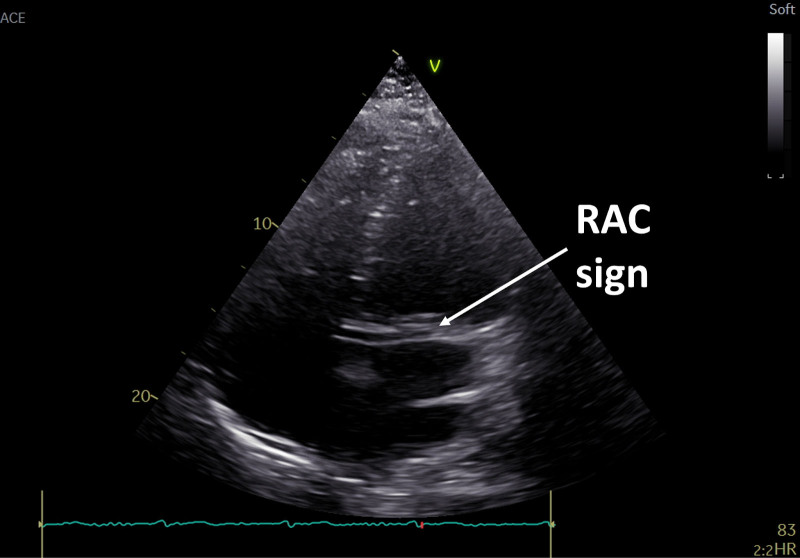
Transthoracic echocardiography images showing the retroaortic anomalous coronary (RAC) sign in a modified four-chamber view.
